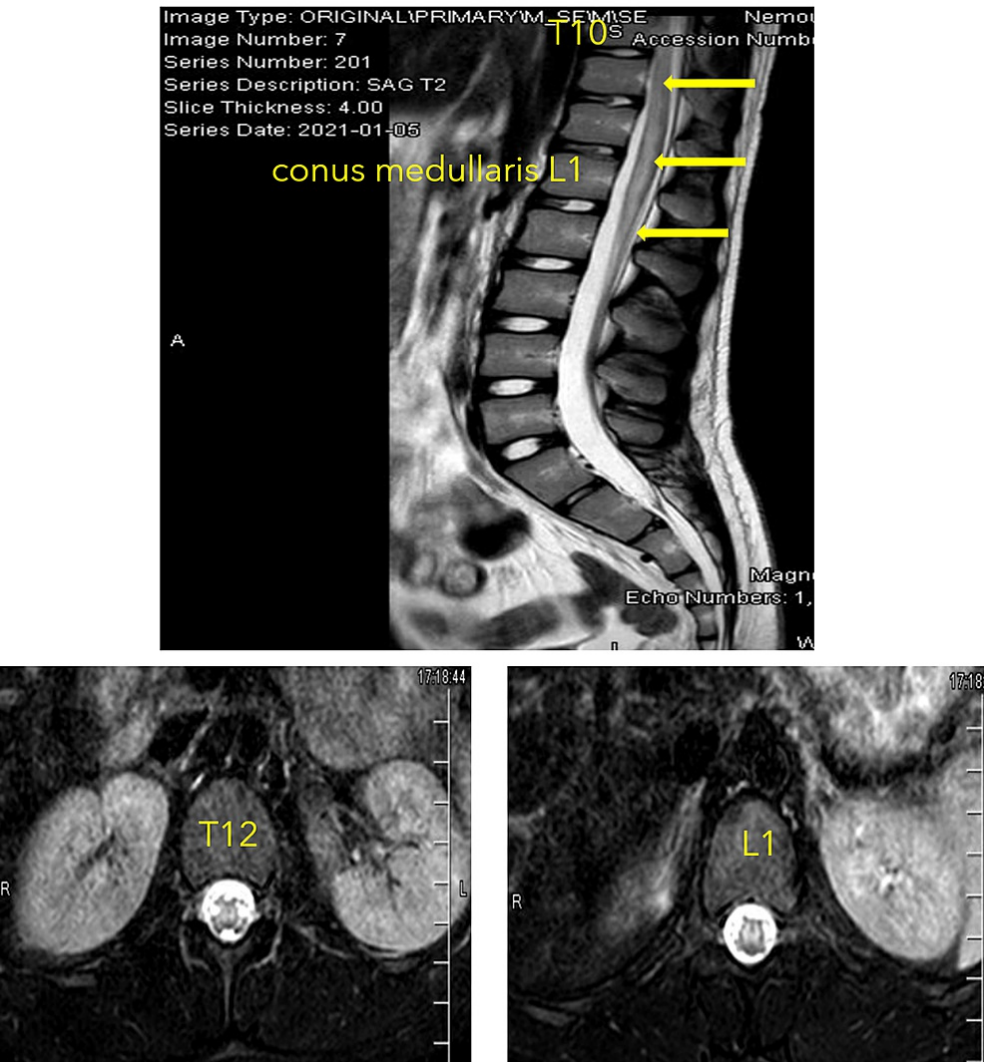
Magnetic Resonance Imaging of the Lumbar Spine There are abnormal T2/STIR signals (arrows) that extend from T10 to the level of conus medullaris. The high-intensity abnormal signals appear to terminate at L2.
